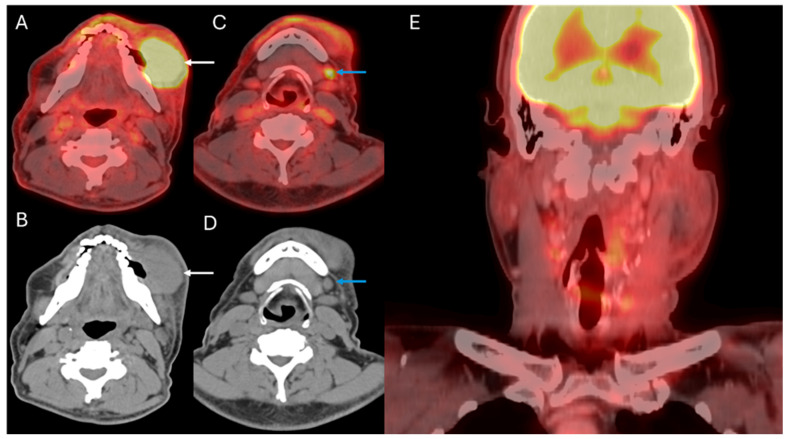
Staging and treatment response evaluation: 62-year-old male with T4aN2b oral cavity squamous cell carcinoma. Axial fused PET/CT (A,C) and axial CT (B,D) of the staging 18F-FDG PET/CT demonstrate the primary tumor involving the left buccal mucosa (white arrows) and left submandibular nodal metastases (blue arrows). Coronal fused PET/CT (E) of the restaging PET/CT after induction chemotherapy, buccal excision, and left neck dissection: adjuvant radiation therapy demonstrates post-treatment changes without suspicious recurrent or residual disease.
